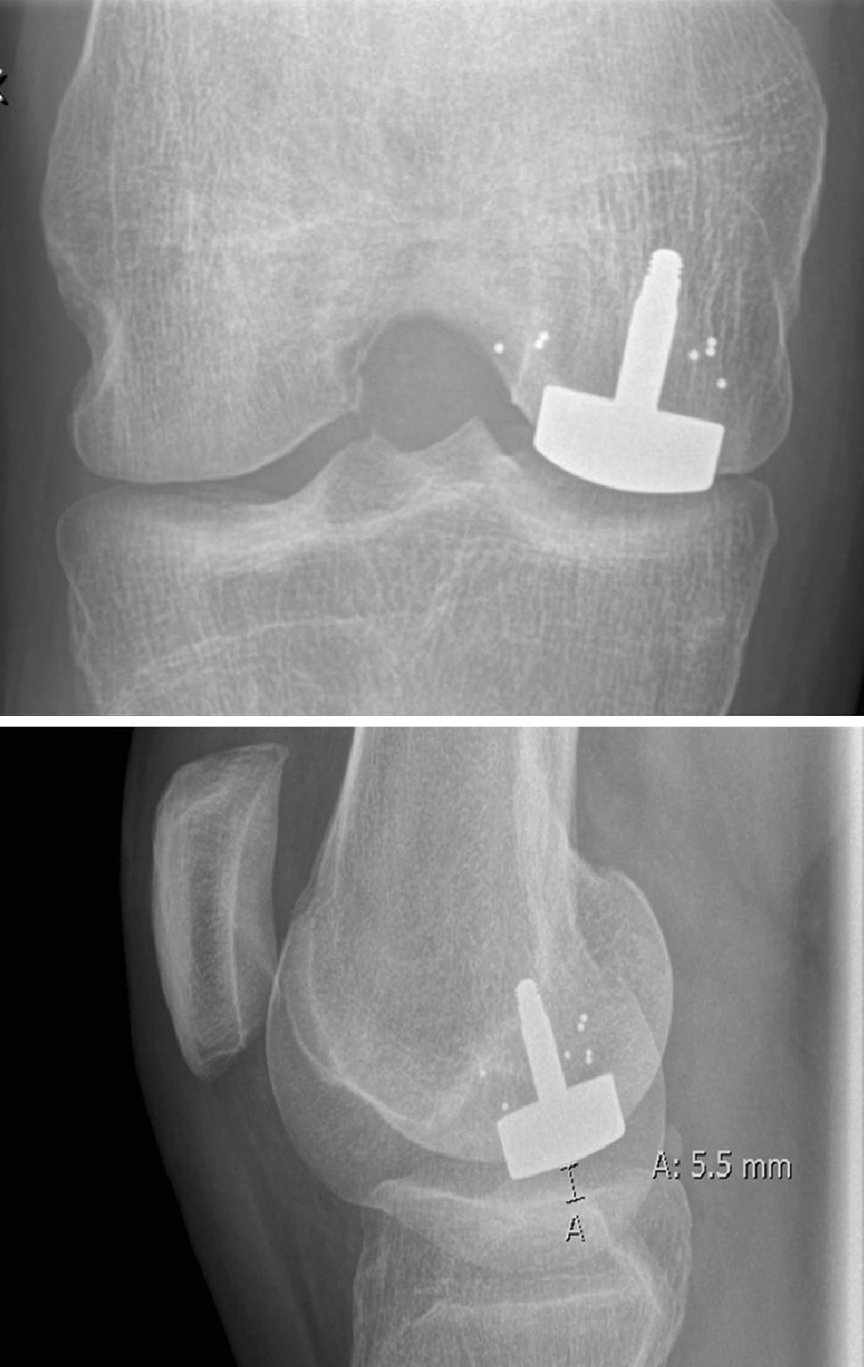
Radiographs, 2 years, patient no. 4
